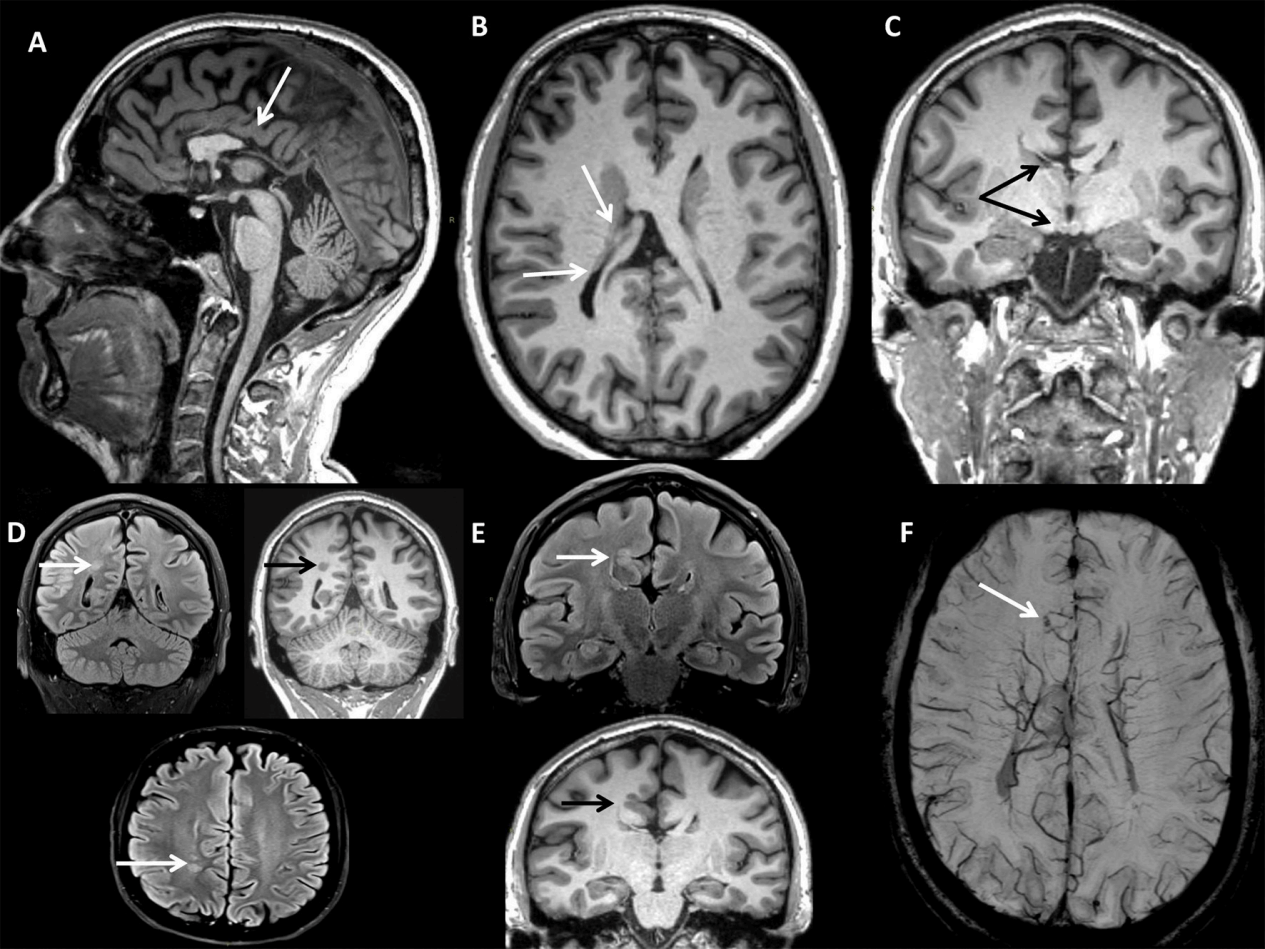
BRAIN MRI (CASE 1): (A) Sagittal 3D Magnetization Prepared Rapid Acquisition Gradient Echo (MPRAGE) T1-w with axial (B), and coronal (C) reconstructions showing partial corpus callosum agenesis, right fornix, and mammillary body hypoplasia, hypogenesis of bilateral lateral ventricles (colpocephaly). (D) Turbo Spin Echo Fluid-Attenuated Inversion Recovery (TSE FLAIR) T2-w axial and coronal (FLAIR T2-w & T1-w) scans showing nodular heterotopic foci. (E) TSE FLAIR T2-w & T1-w coronal scan showing right frontal parasagittal cortical thickening. (F) Susceptibility Weighted Imaging (SWI) axial scan with Maximum Intensity Projection (MIP) reconstruction showing right frontal parasagittal venous drainage abnormality with telangiectasia.
